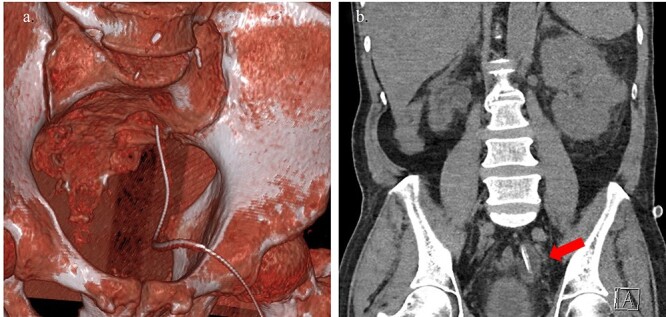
(a) 3D-reconstruction CT image shows the suprapubic catheter; (b) frontal CT image shows the right atrophic kidney, as well as the insertion of the suprapubic catheter from the bladder to the left ureter with distal hydroureter and the inflated balloon (red arrow).
